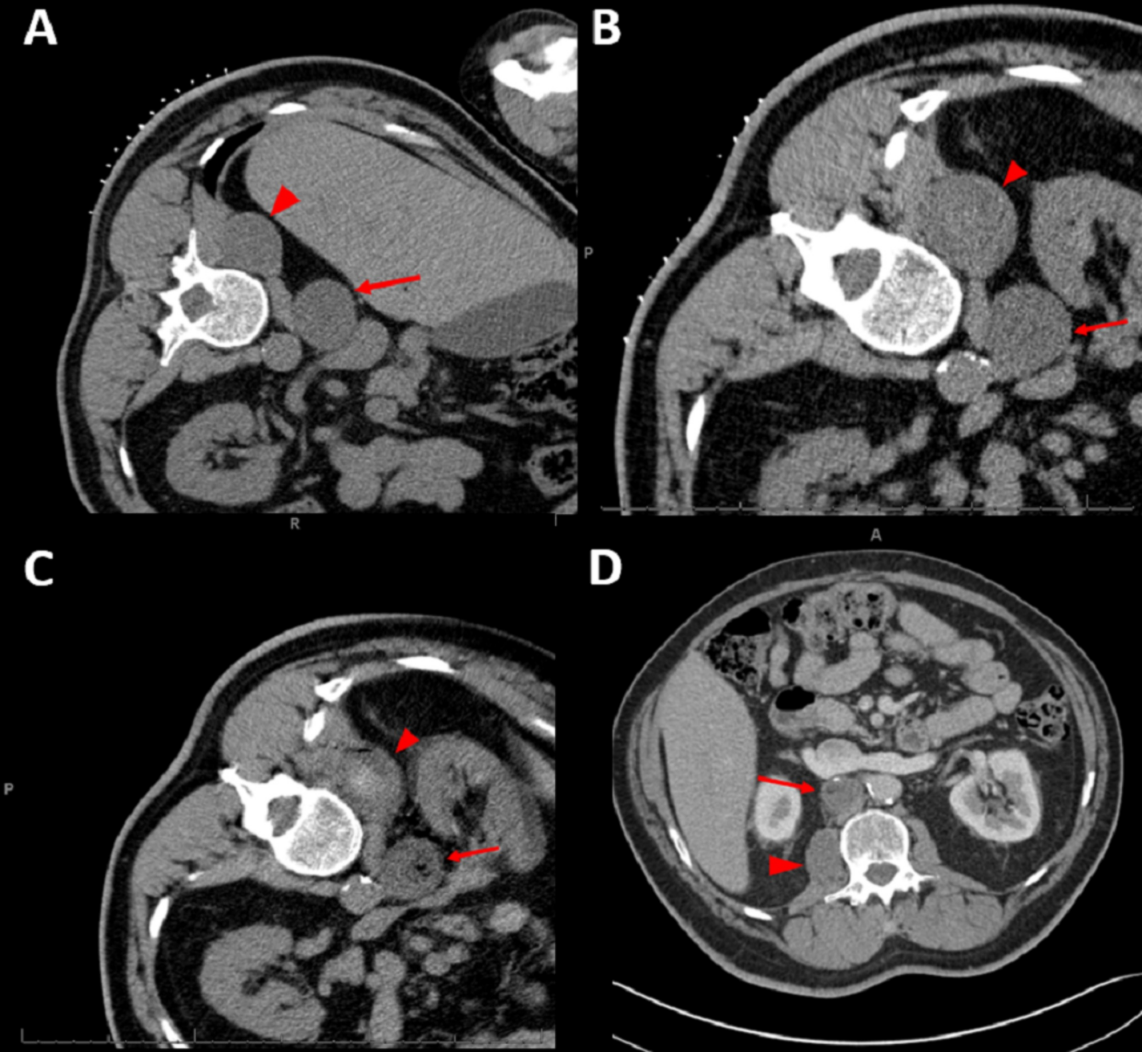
Pre and post-ablation images of the two retroperitoneal neurofibromas 1A. The CT scan shows the two lesions at the time of presentation/biopsy. 1B. The CT scan at the time of the RFA three years after presentation. 1C. Immediate post-ablation CT images show the anticipated changes, with a small amount of air within the anterior lesion (arrow) and a hyperdense hemorrhage within the paraspinal lesion (arrowhead). 1D. Four years post-ablation. Both lesions show a sustained decrease in size. (The anterior lesion is denoted with the arrow, and the paraspinal lesion is denoted with the arrowhead) CT: computed tomography; RFA: radiofrequency ablation
